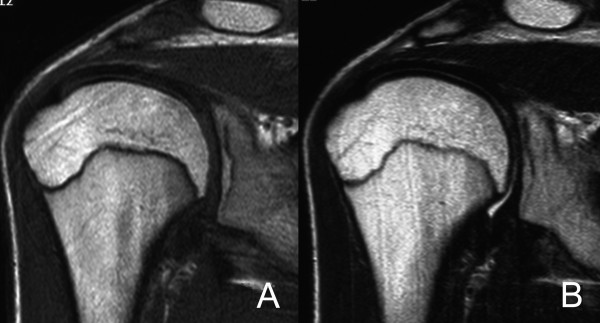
A coronal oblique T1-weighted magnetic resonance image of the shoulder (2008-6-30) (spin-echo sequence with a repetition time of 500 msec and an echo time of 13 msec) shows normalization of the epiphyseal marrow signal (A), and a coronal oblique T2-weighted magnetic resonance image of the shoulder (2008-6-30) (spin-echo sequence with a repetition time of 3762 msec and an echo time of 90 msec) shows normalization of the epiphyseal marrow signal (B).
